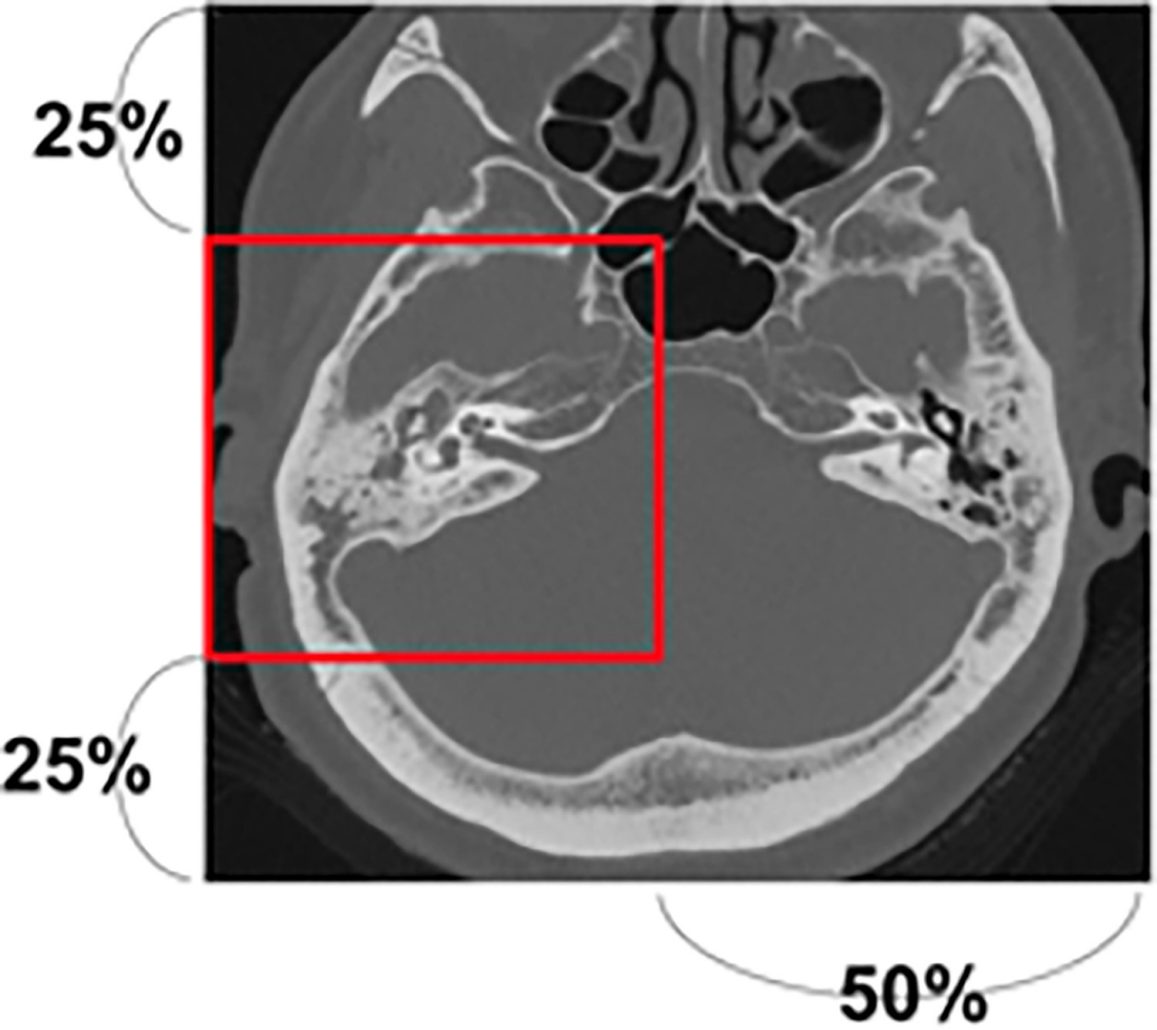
CT images used. We evaluated the accuracy of prediction using 25% partial CT images that contain lesion areas cropped from the vertical center 50% and horizontally left or right 50%.
